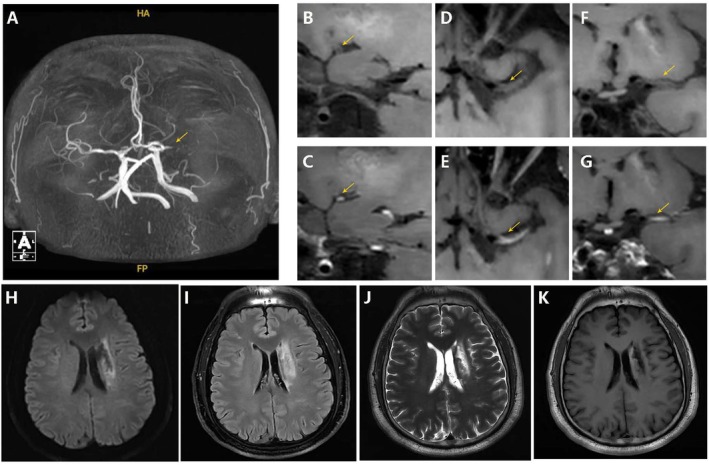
Two months after the onset of the patient's condition, high‐resolution 3 T intravascular wall imaging revealed occlusion in the left M1 segment with no distal blood flow. TOF images (A), MRI sagittal (B), axial (D), and coronal (F) views, as well as enhanced images (C, E, G), demonstrated significant vascular wall enhancement. DWI (H), Flair (I), T2 (J), and T1 sequences (K) on non‐contrast MRI indicated recovery of the left basal ganglia infarct area without evidence of new‐onset cerebral infarction.
